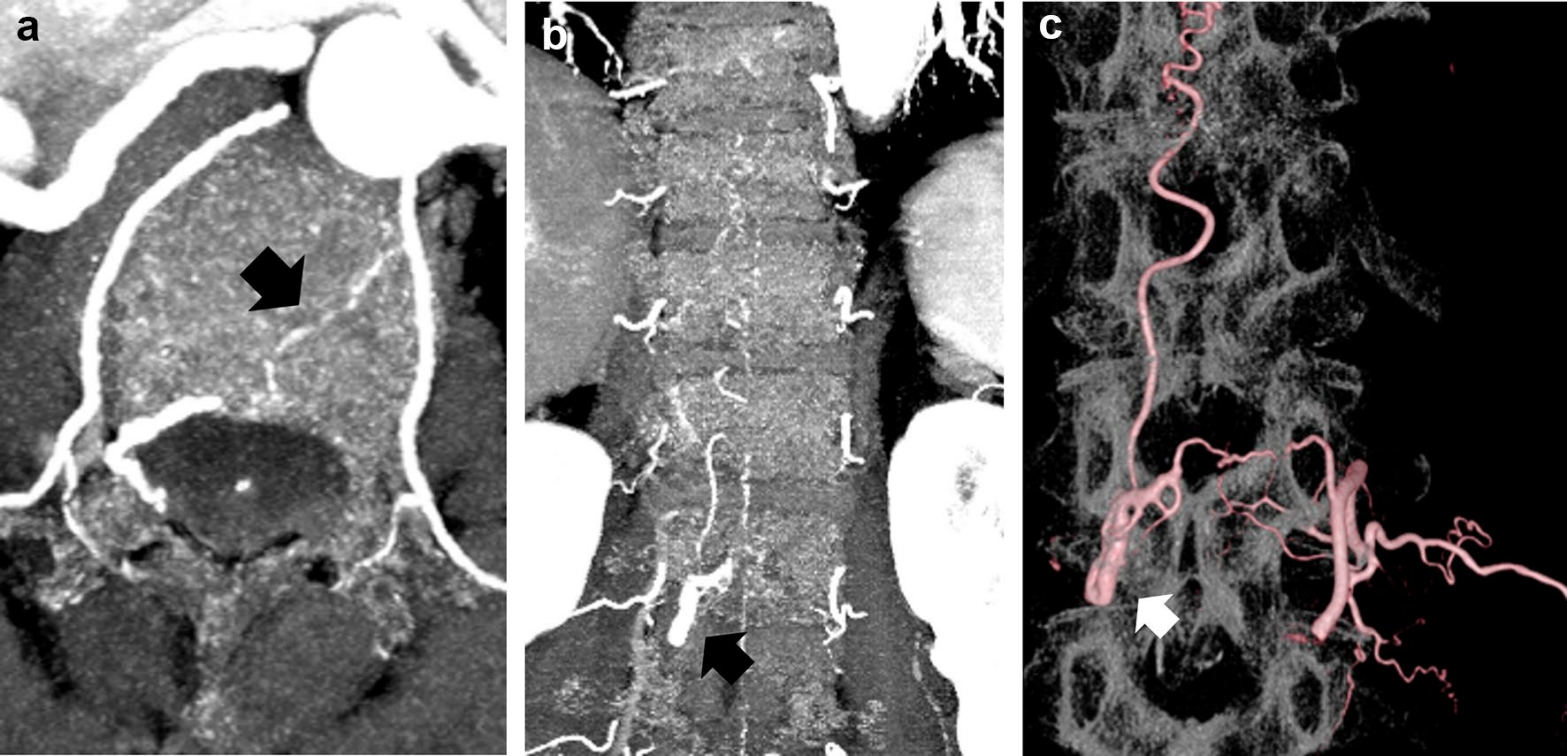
Spinal epidural arteriovenous fistula (AVF). A case of a female patient in her 60 s with complaints of numbness, coldness, and stiffness of both lower extremities for several months. On magnetic resonance imaging (MRI), the T1-weighted images (T1WI) and T2-weighted images (T2WI) showed extensive edema in the spinal cord and multiple flow voids on the surface of the spinal cord. The PCD-CTA (axial image: kernel Qr60) shows the ventral somatic branch (arrow), which branches from the L1 segmental artery, internally traverses the vertebral body, and joins into the epidural shunted pouch. The epidural shunted pouch continues with the radiculomedullary vein and refluxes into the posterior spinal veins. Comparison of the PCD-CTA images (a, b) with selective angiography coronal view (c) shows that the L1 segmental artery, the ventral somatic branch (a, arrow), the shunted pouch (b, arrow), and the ascending retrograde radiculomedullary vein were almost equally well delineated. *PCD-CTA* photon-counting detector-computed tomography angiography
